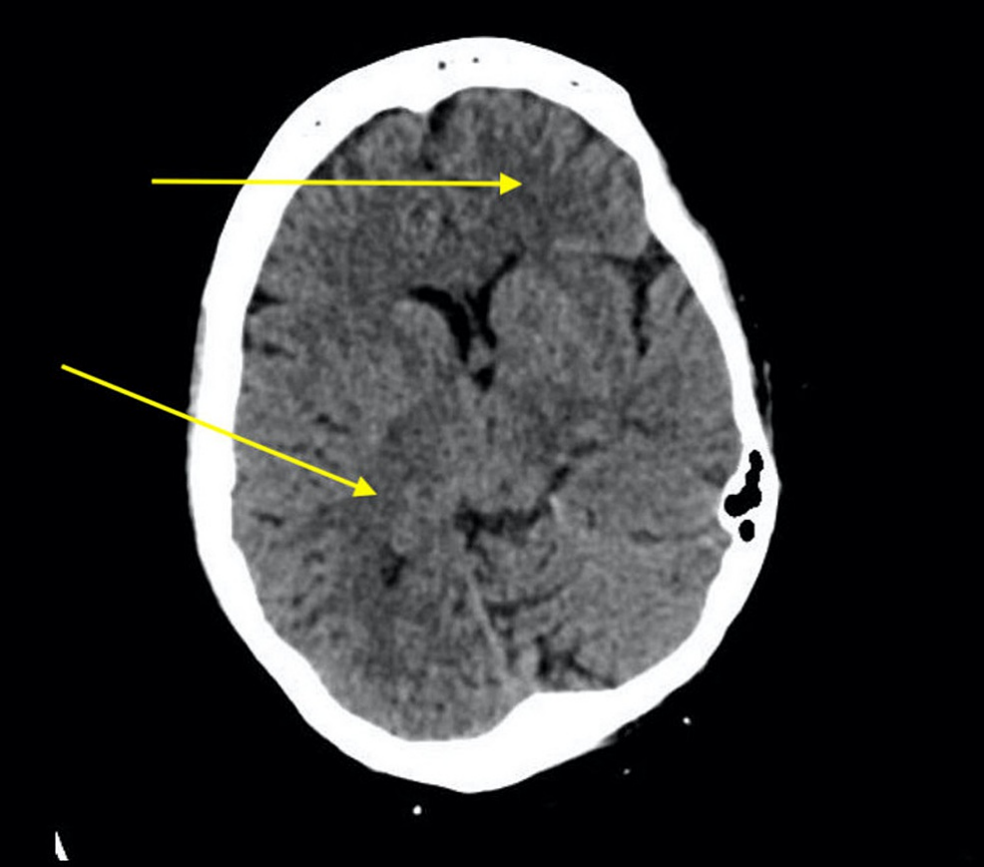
Final non-contrast CT of the brain Yellow arrows show the labeled new hypodensity regions in the frontal lobe and white matter hypodensities in the right high parietal lobe.
